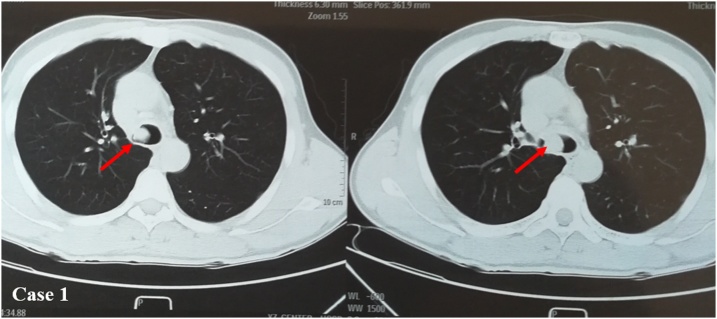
Case 1. Chest CT shows tumor infiltration between carina and the right main bronchus (see the arrow).
